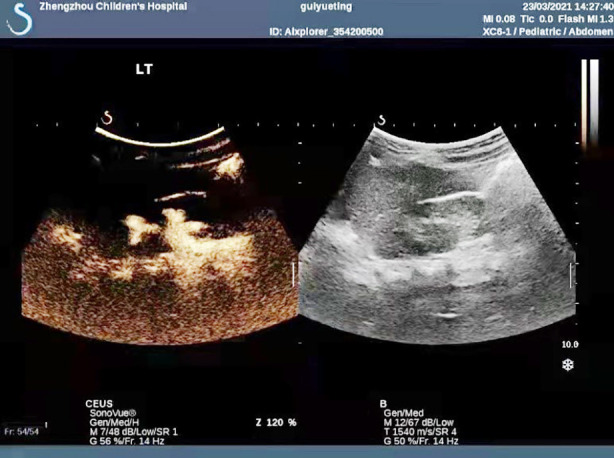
Image after contrast-enhanced cystosonography.
